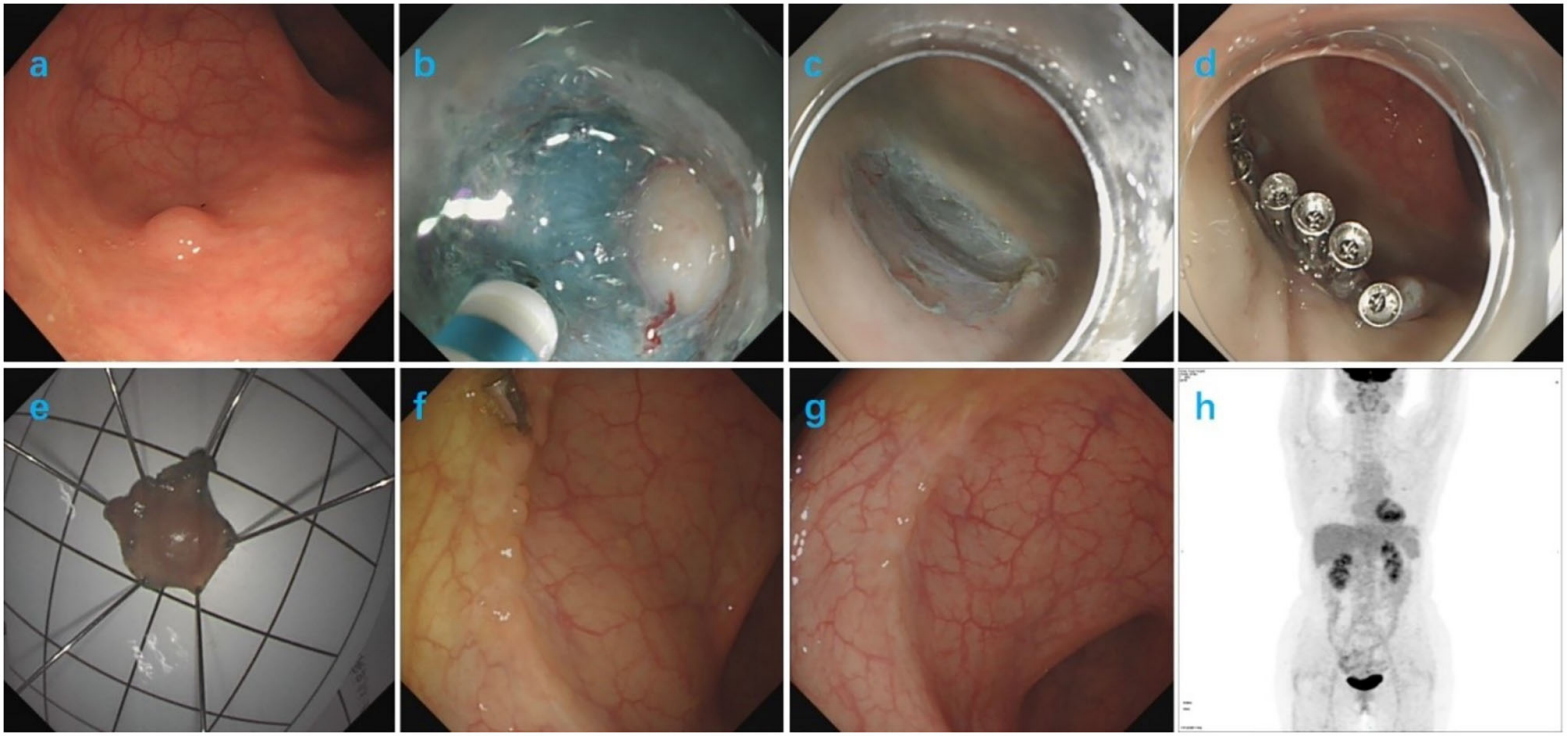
(a) Colonoscopy showed a slightly yellowish, submucosal tumor-like 5-mm protrusion in the rectum. (b–e) The procedure of ESD. (f) Three months after ESD, a follow-up colonoscopy showed complete resolution of the elevated lesion and a residual titanium clip. (g) Nine months after ESD, a follow-up colonoscopy showed complete resolution of the elevated lesion. (h) PET/CT revealed no evidence of malignancy in the whole body after ESD.
